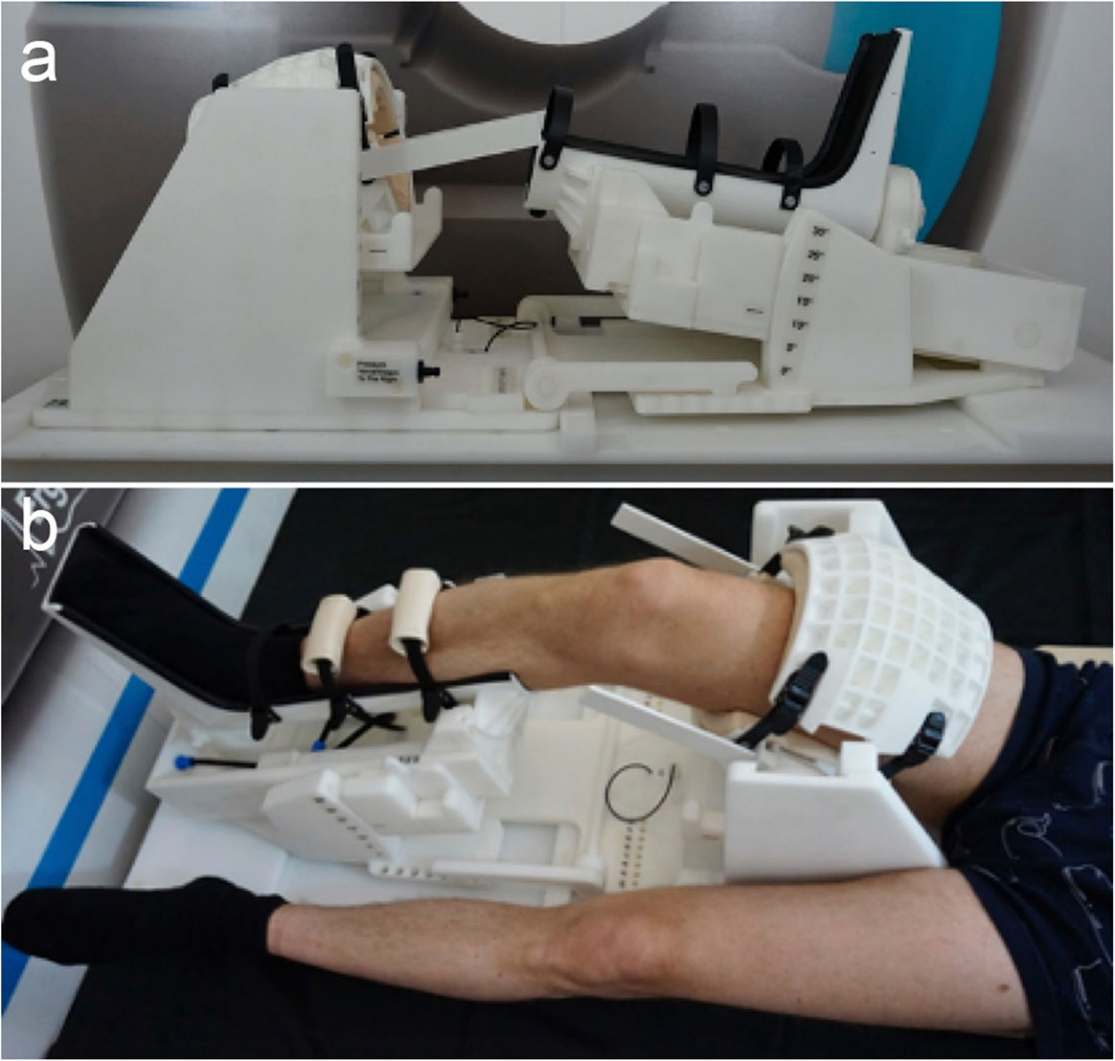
a MRI-safe, pneumatically driven knee fixation device. The “boot” of the device may be rotated upwards, to exert pressure against the posterior aspect of the calf, with the thigh being firmly anchored. This creates a Lachman test-like scenario in which the anterior cruciate ligament is strained. b Subject positioning within the device
